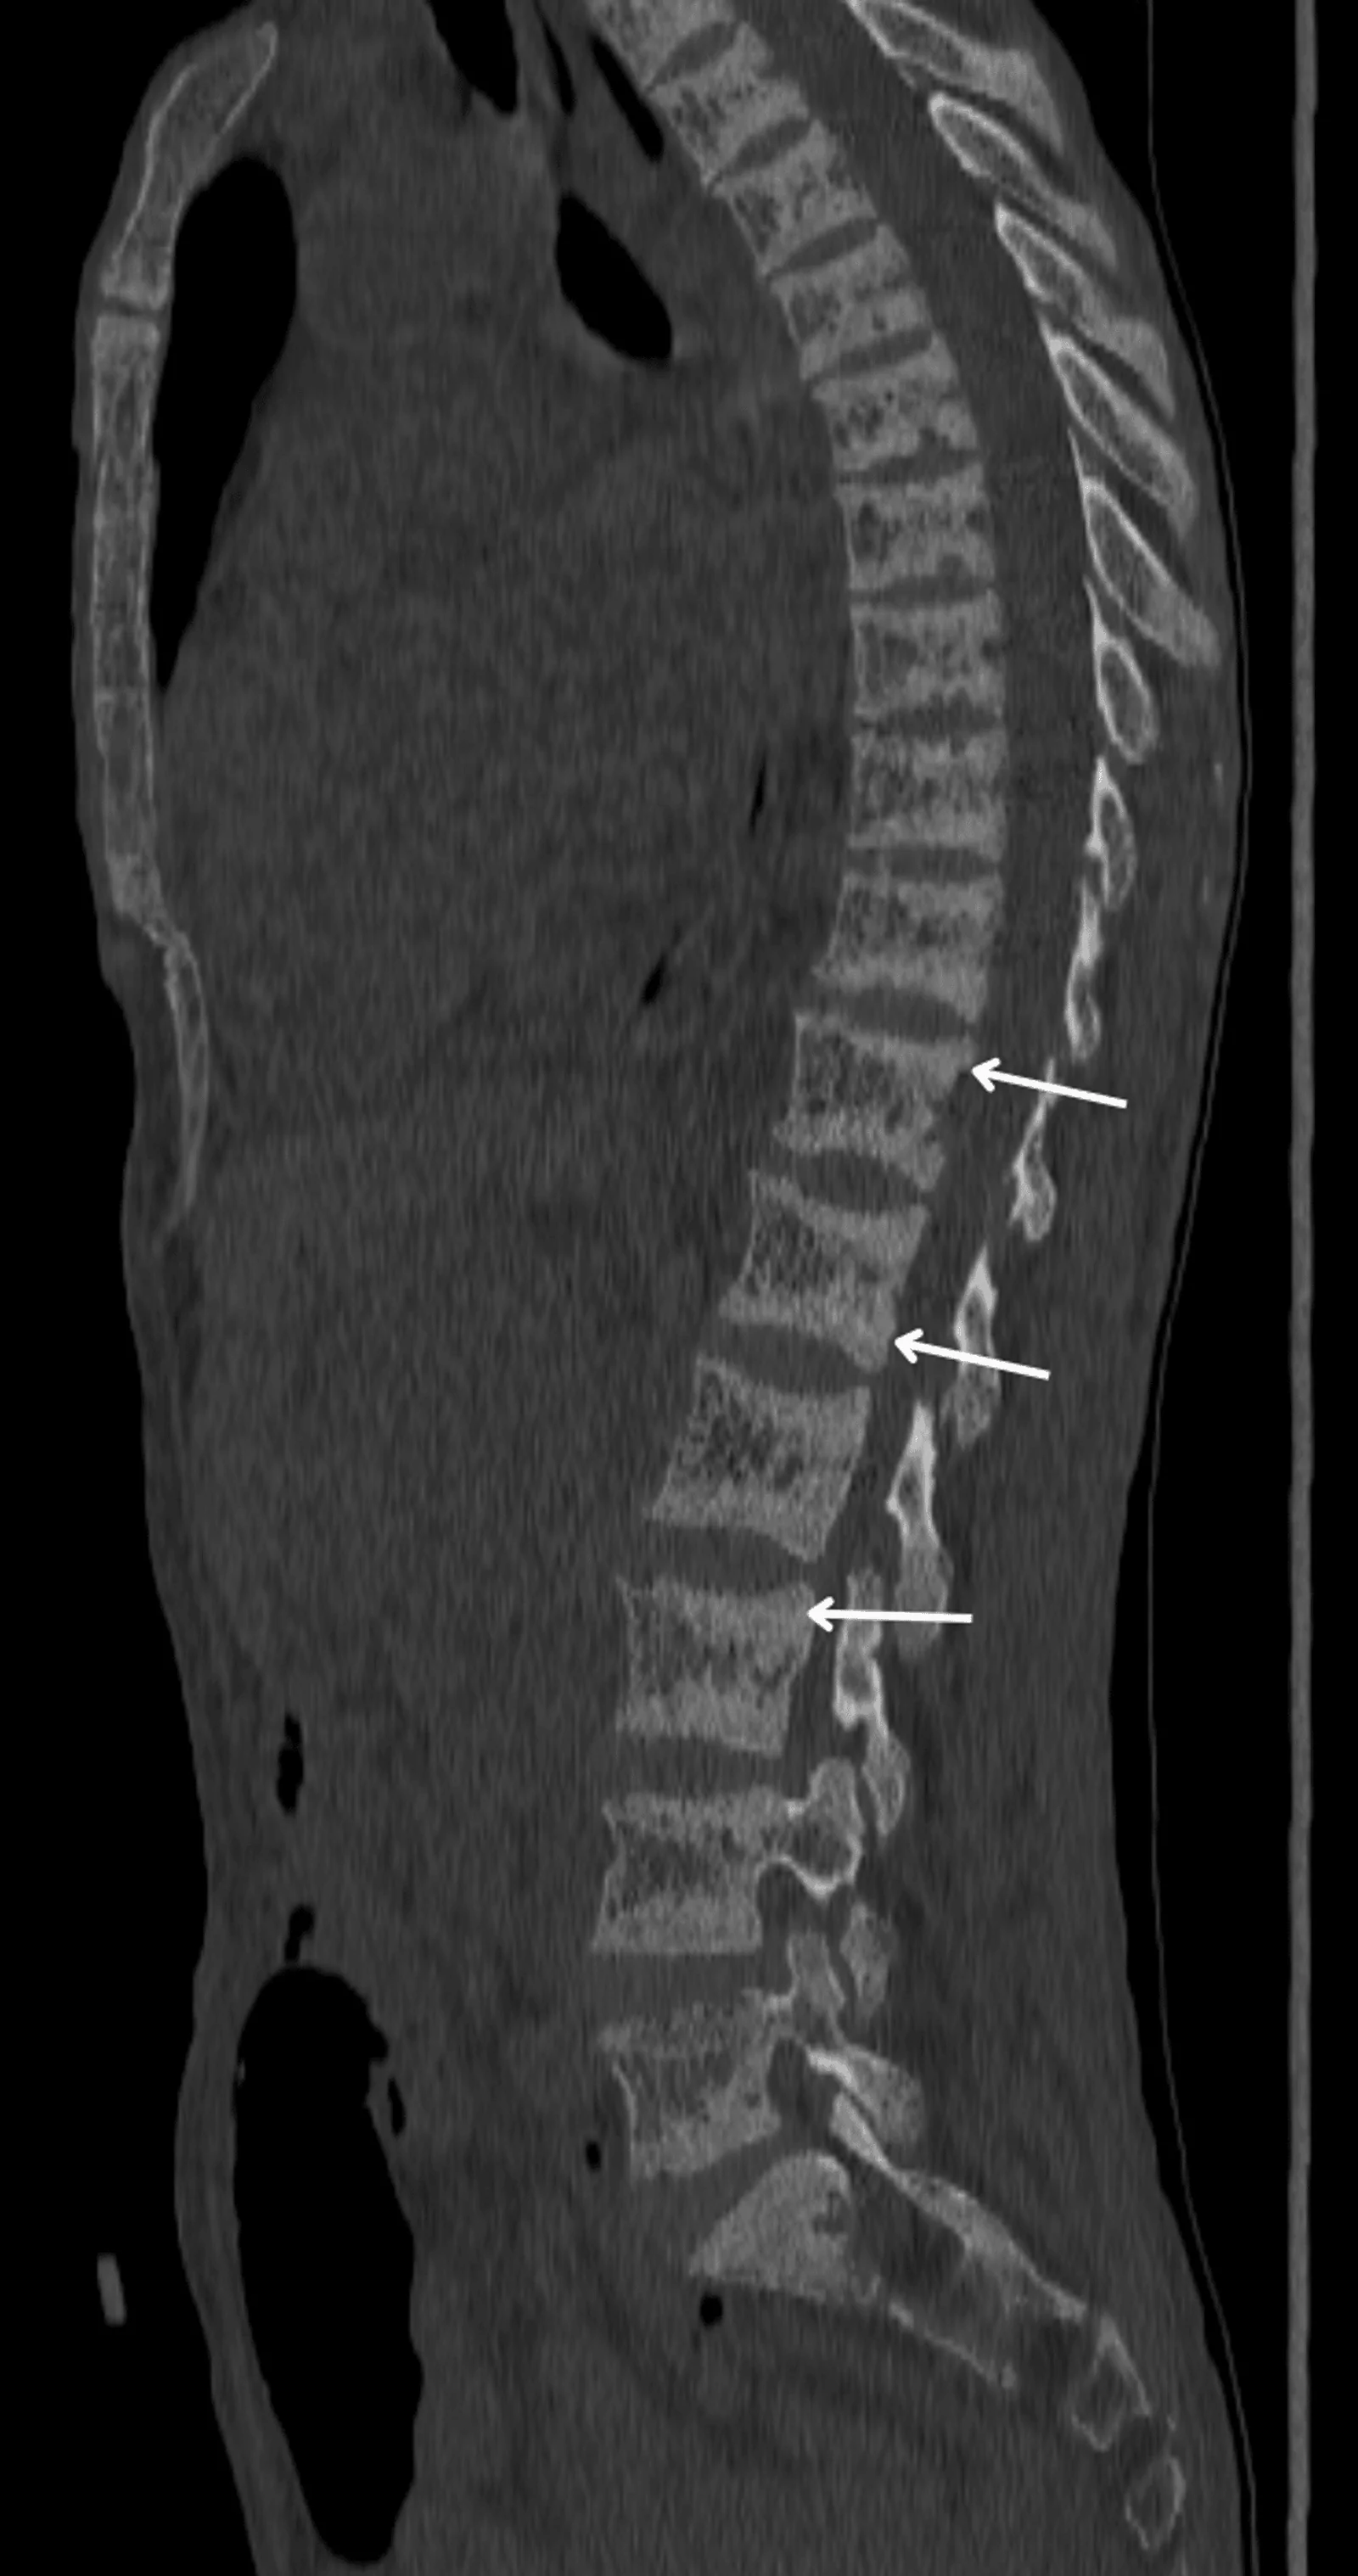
Sagittal bone-window CT image showing diffuse sclerosis of the superior and inferior vertebral endplates with relative central lucency, producing the characteristic rugger-jersey spine appearance (arrows), consistent with renal osteodystrophy secondary to chronic kidney disease.
